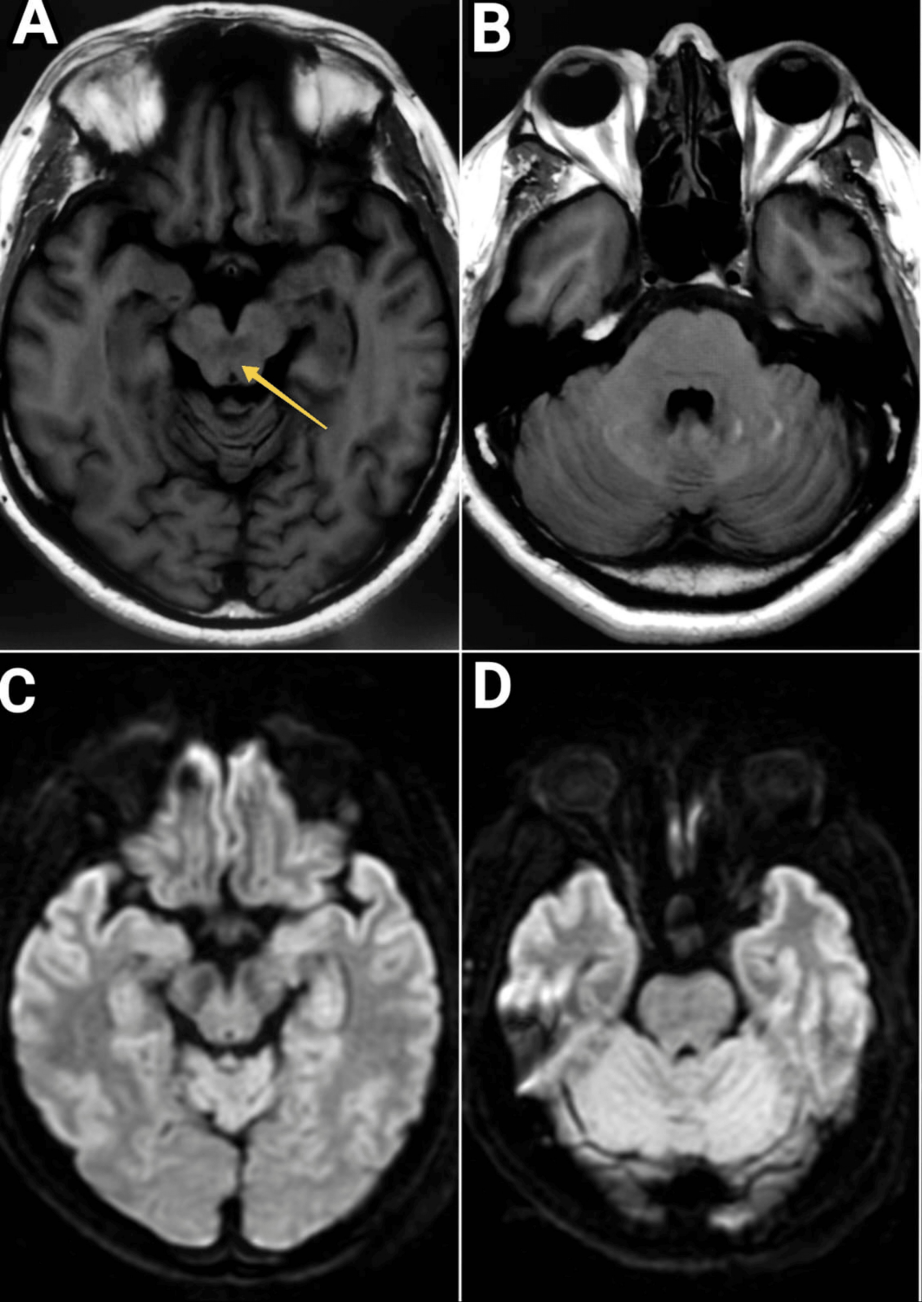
MRI brain shows a lesion-free MLF in the midbrain and pons. (A) T2 FLAIR at the level of midbrain, (B) T2 FLAIR at the level of pons, (C) DWI at the level of mid brain, and (D) DWI at the level of pons. FLAIR: fluid-attenuated inverse recovery; DWI: diffusion-weighted imaging; MLF: medial longitudinal fasciculus.
